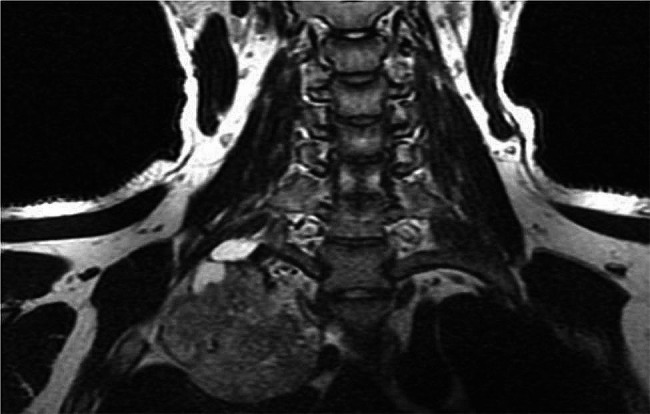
T2-weighted magnetic resonance images show a coronal view
